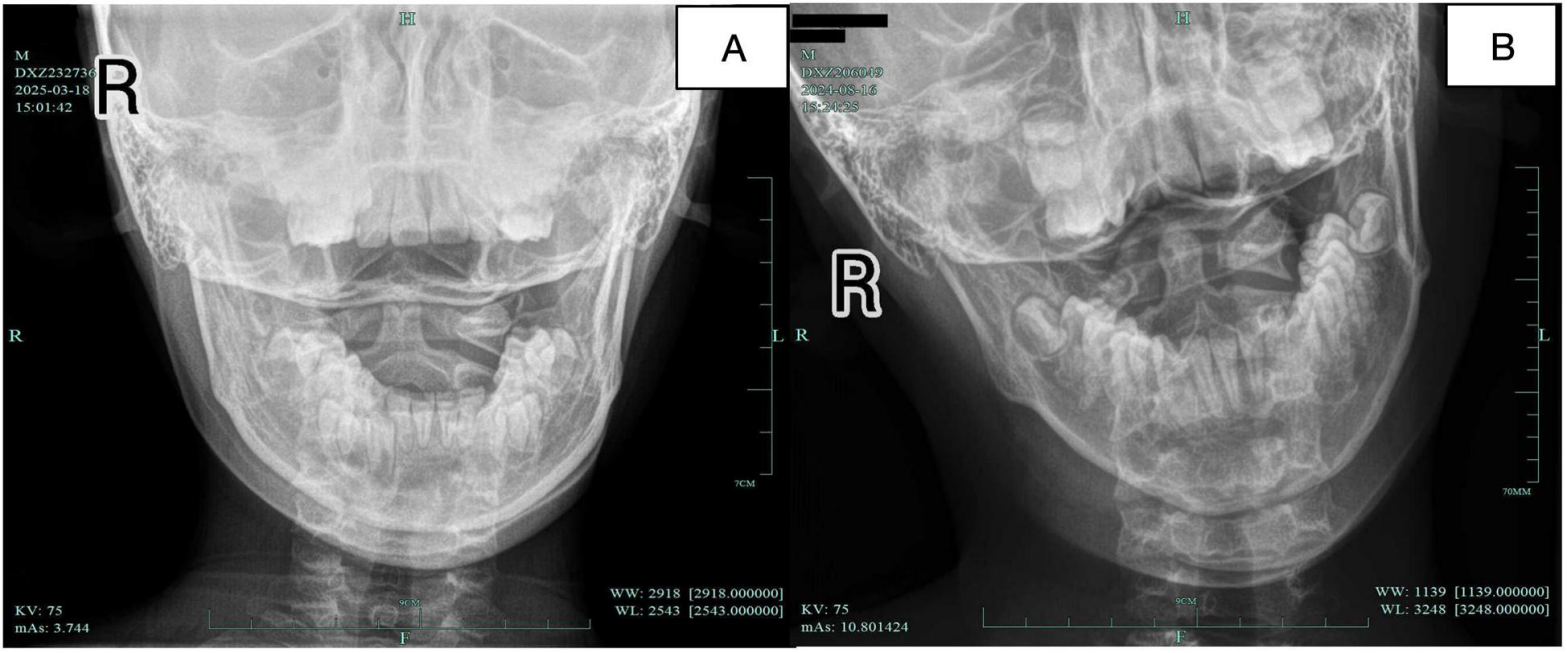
(A) Normal atlantoaxial joint x-ray image; (B) AARS x-ray image.
